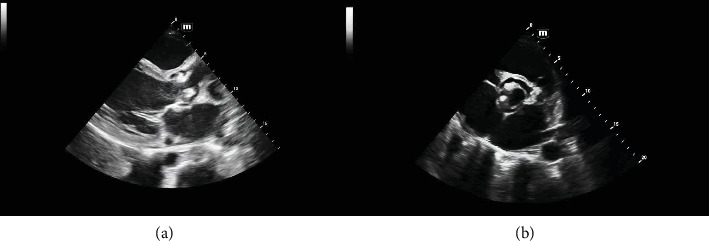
Transthoracic echocardiography revealing. (a) Large vegetations attached to both aortic valve leaflets in the long axis view. (b) Bicuspid aortic valve with the vegetation in the short axis view.
